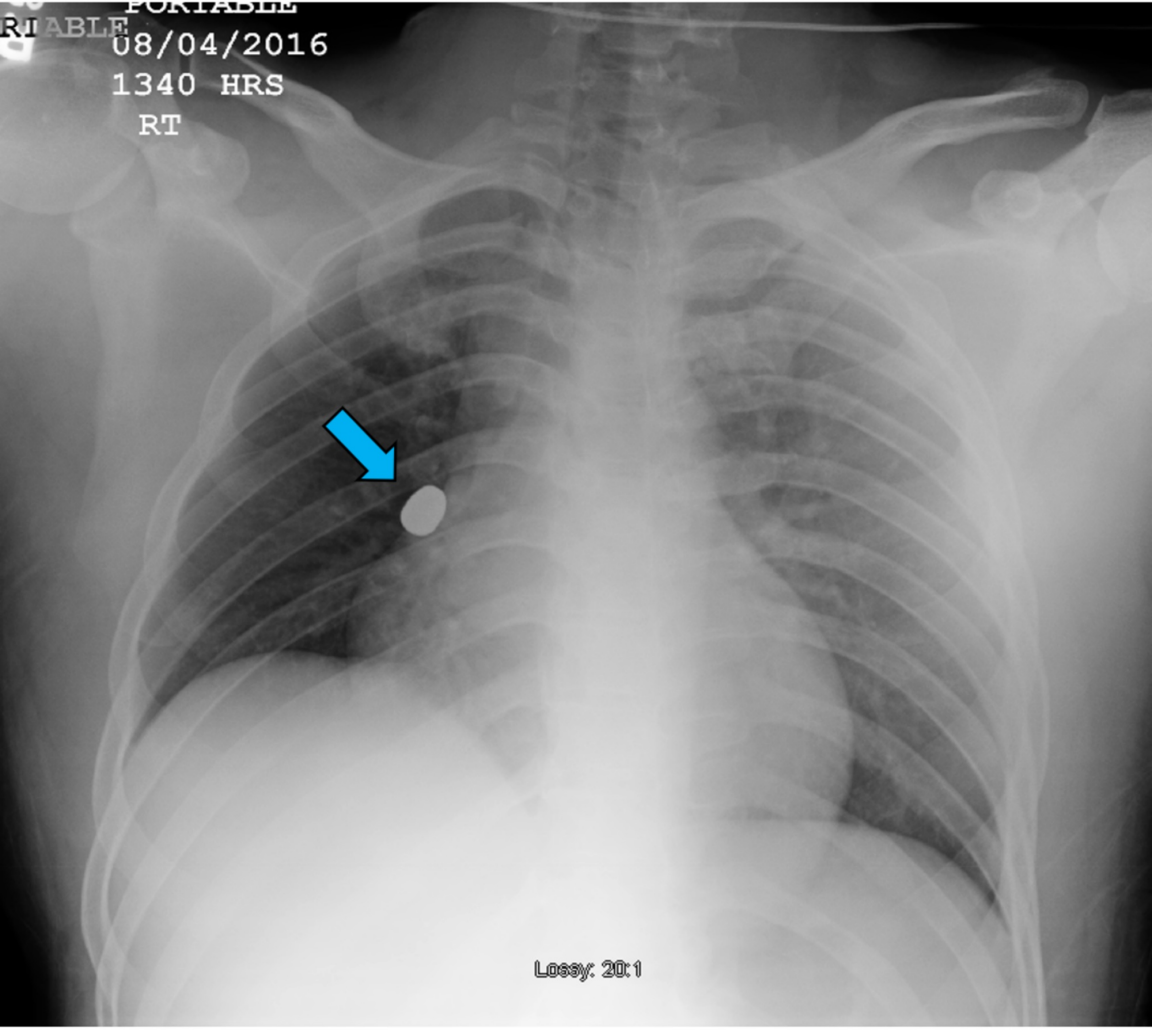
Supine chest radiograph, anterior-posterior view, with the bullet lodged likely in the right atrium (blue arrow).
